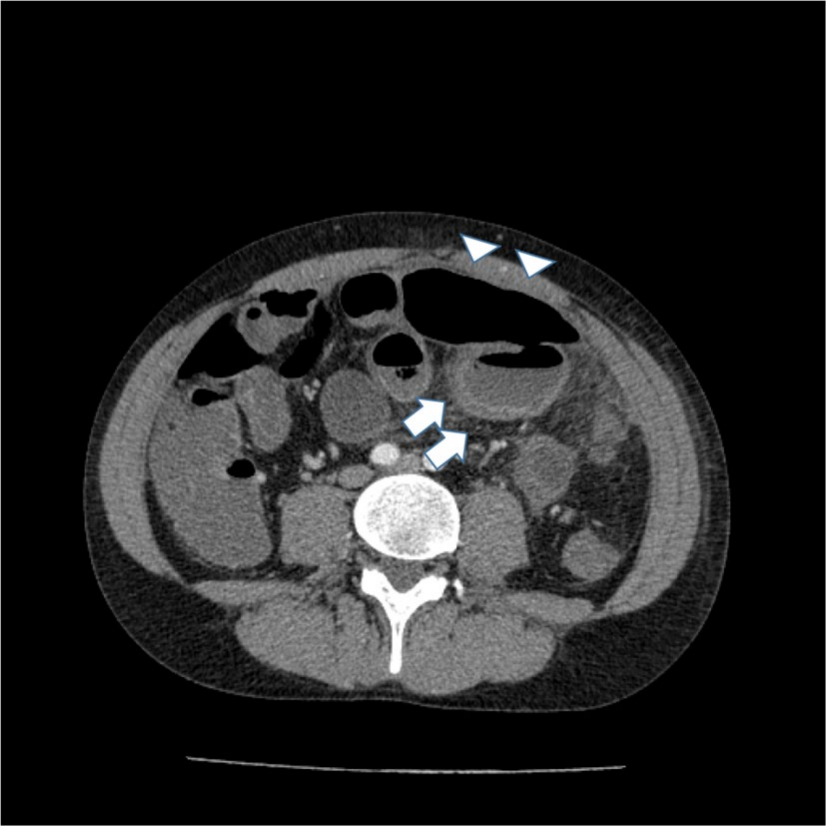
Abdominal computed tomography findings on the third day after patient admission to the hospital for abdominal pain. Wall thickening and thinning of the sigmoid colon (white arrows) and free gas in the abdominal cavity (white triangles) are observed
